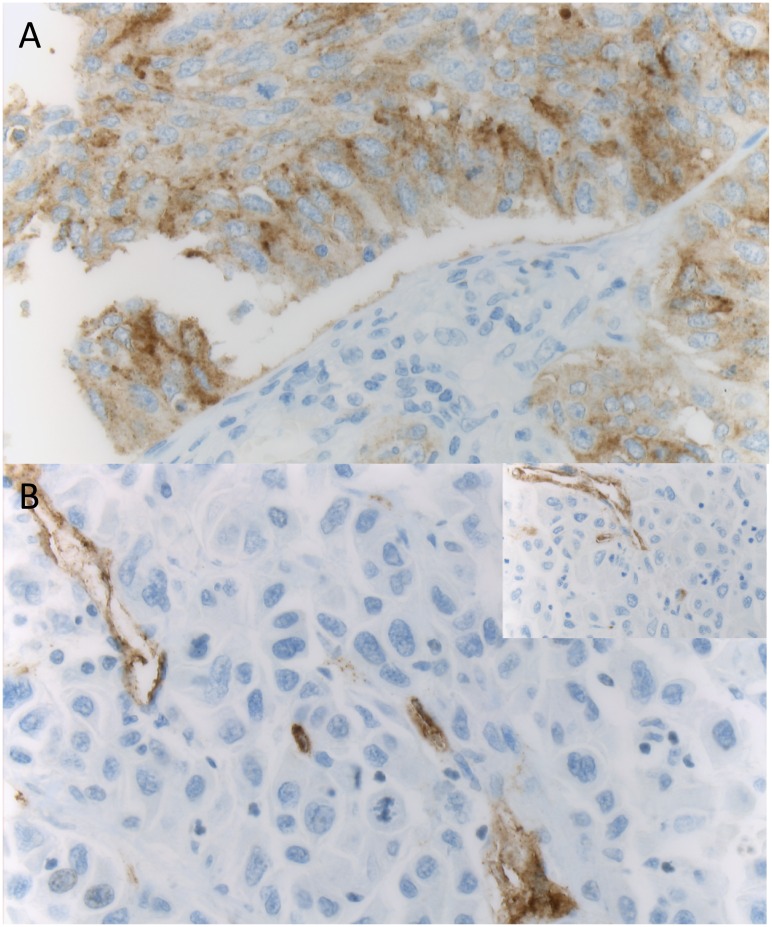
PSMA expression in non-small cell lung cancer (NSCLC). A (100x, anti-PSMA) displays strong PSMA expression in tumor cells. In B (100x, anti-PSMA), neovascular PSMA expression is shown. Neovasculature was identified by CD34 coexpression (insert, anti-CD34).
